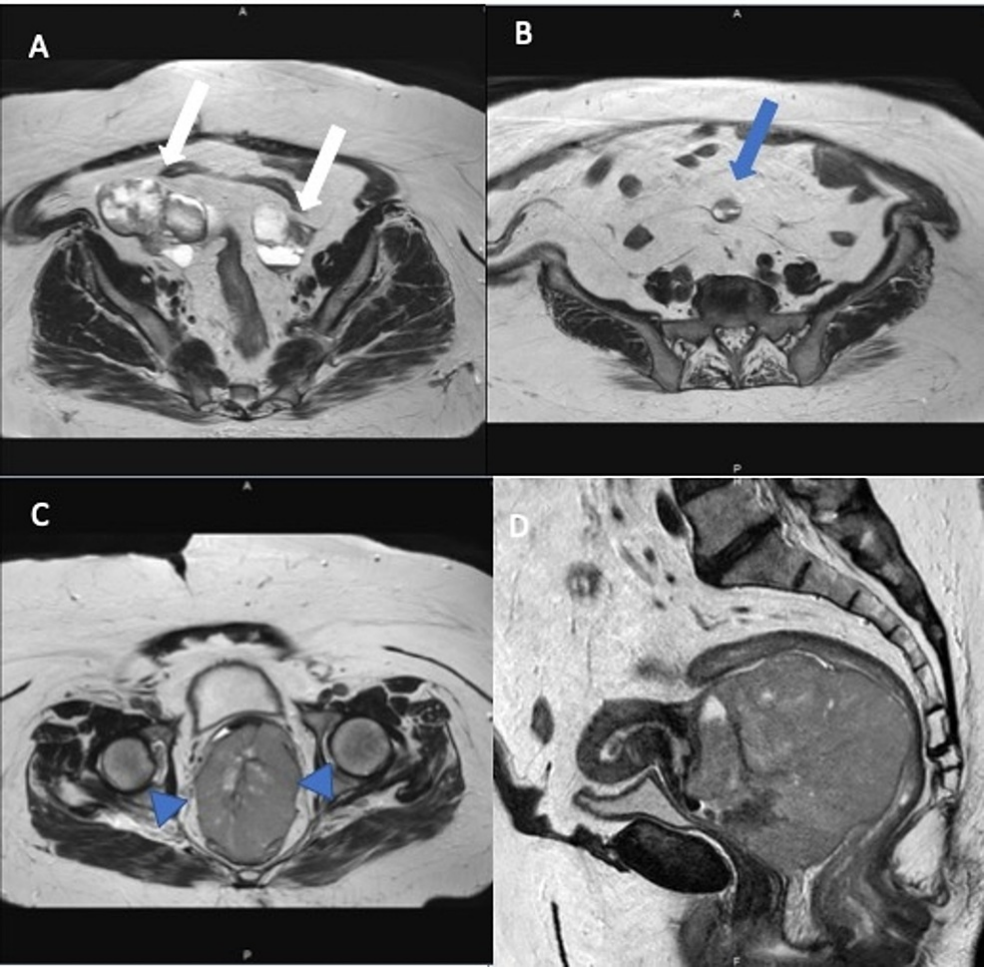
Pelvic MRI images. A: Axial T2-weighted image shows multicystic neoplastic masses (white arrows) with solid components in both ovaries. B: A peritoneal implant (blue arrow) with similar characteristics to the ovarian lesions is seen in the peritoneal cavity. C and D: T2-weighted images of the uterine cervix in the axial and sagittal plane reveal the bulky cervical tumor. The cervical stromal ring is well preserved (arrowhead). However, the anterior rectal wall continuum is not very well seen.
